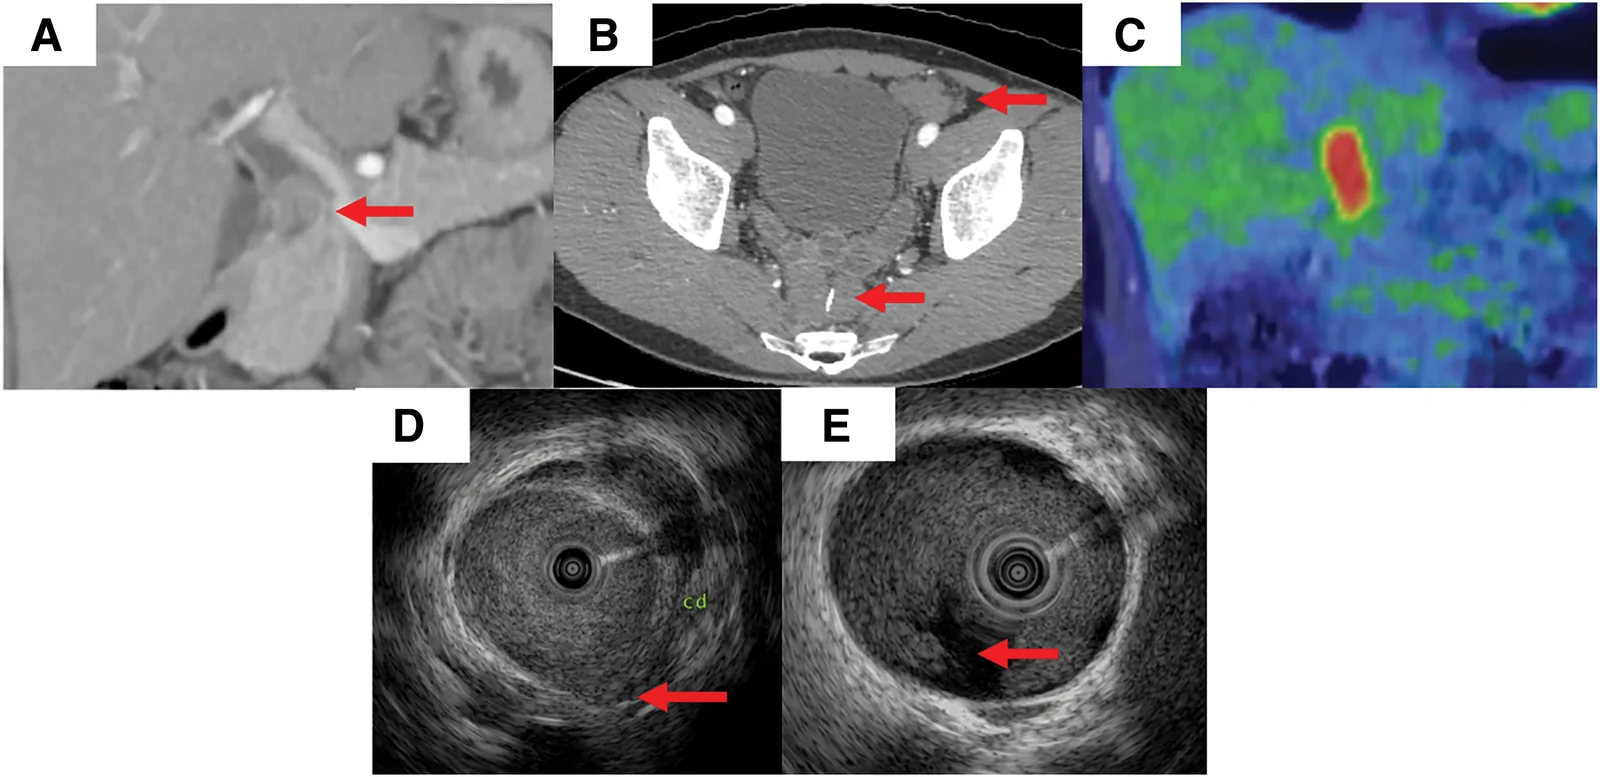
(A) Contrast-enhanced CT showing a mass lesion in the common bile duct. (B) Contrast-enhanced CT demonstrating local recurrence in the rectum and pelvic recurrence. (C) FDG-PET/CT depicting abnormal uptake in the common bile duct (SUVmax 9.1). (D) EUS showing an irregular mucosal surface within the common bile duct and a hypoechoic mass filling the lumen. (E) EUS of a protruding mass lesion within the common bile duct. EUS, endoscopic ultrasonography
